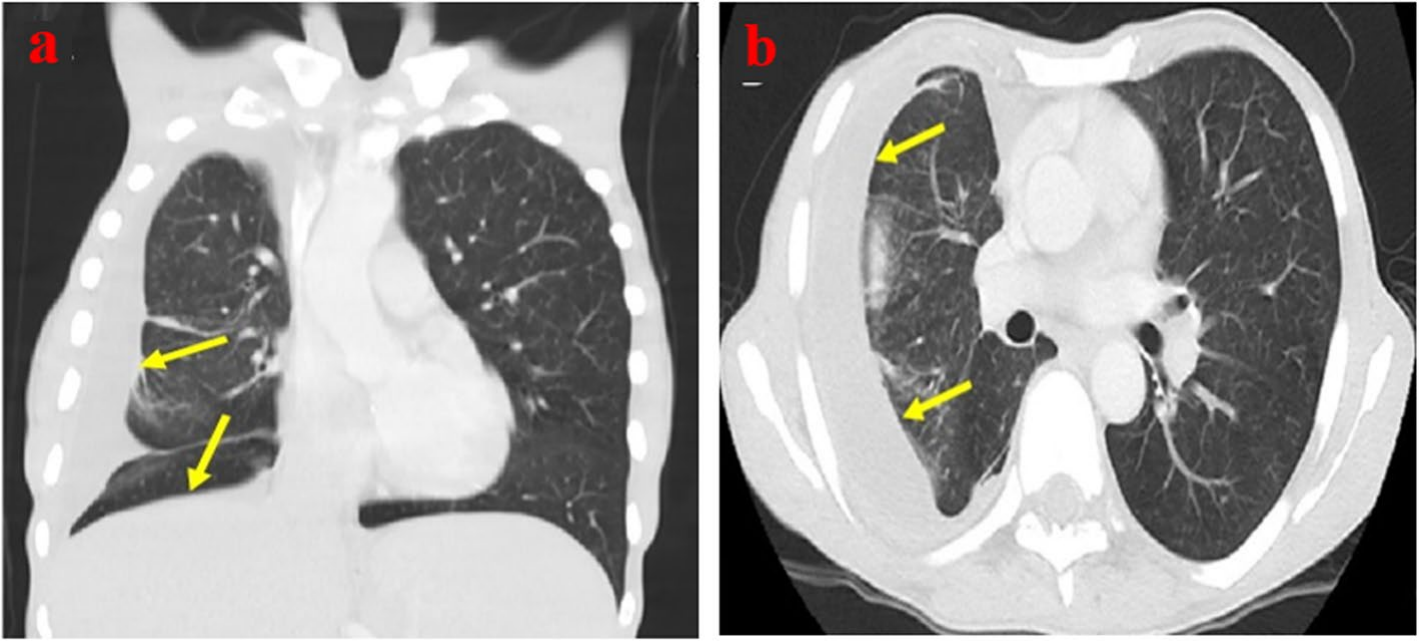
CT scan chest with coronal (a) and transverse (b) sections of lungs showing right-sided pleural effusion
